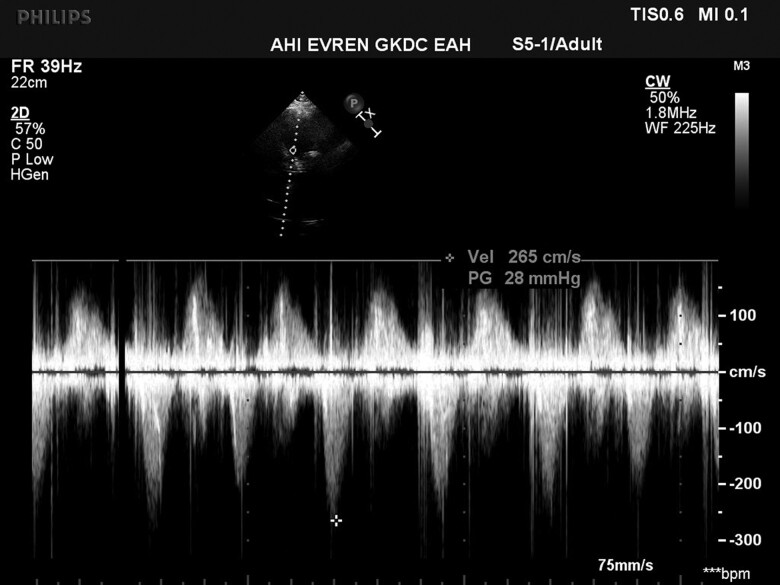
Doppler echocardiographic measured maximal gradient of 28 mmHg in the left ventricular outflow tract after the kissing balloon manoeuvre.
